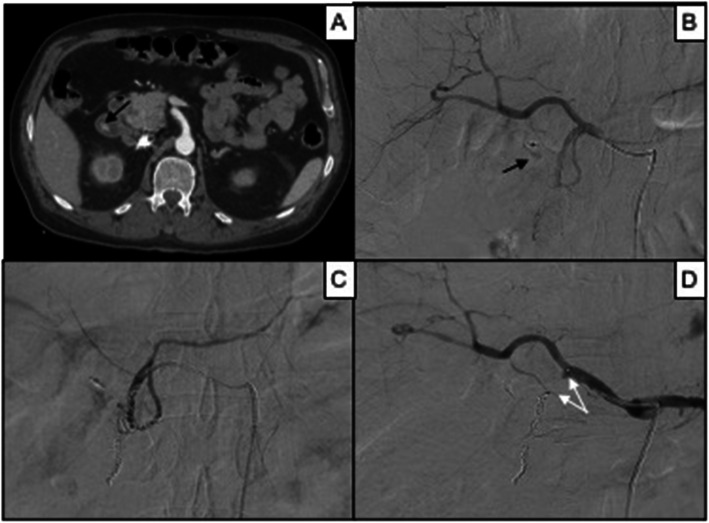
67 years old male affected by bleeding duodenal ulcer; previous attempts to manage the patient endoscopically failed. In A, a contrast-enhanced CT scan in arterial phase shows active intraluminal bleeding (black arrow); in B, DSA confirms active bleeding (black arrow) from the gastroduodenal artery; in C, DSA shows uneffective coiling of the gastroduodenal artery with blood flow continuing into the vessel; in D, DSA shows complete gastroduodenal artery occlusion after release of MVP-7 (white arrows) in correspondence of the origin of the vessel (target vessel caliper 4.1 mm)
